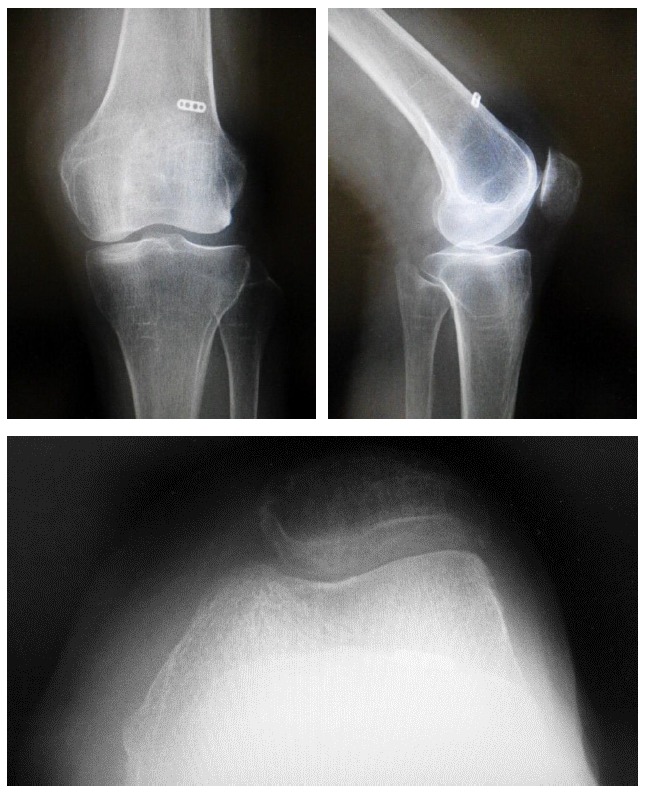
Postoperative roentgenogram taken after medial patellofemoral ligament reconstruction.
